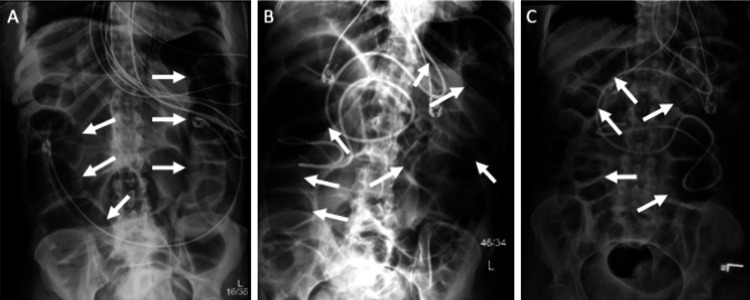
Abdominal X-ray imaging of the patient throughout the hospital course. Arrows demonstrating the level of colonic distension and subsequent compression (A) Acquired several days after admission into the medical intensive care unit, demonstrating marked gaseous extension of the colon; (B) Worsening gaseous distention of the small and large bowel, correlating with hyperactive abdominal and pseudo-obstruction. (C) Decreased abdominal distention after nasogastric and rectal tube placement
